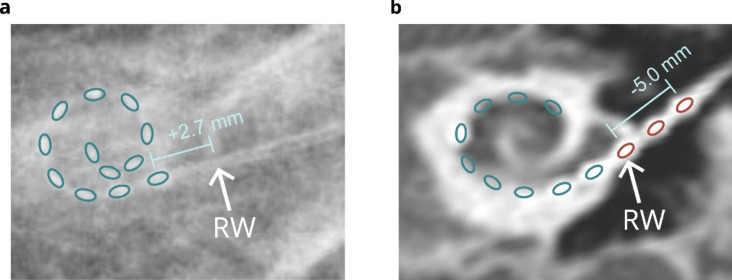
Comparison of postoperative radiographs showing the insertion depth of CI electrodes implanted in the left ear. Linear insertion depth into the cochlea was measured along the electrode array starting from the round window (RW), as indicated by the white arrow. a Plain radiography one day after the surgery. All electrodes are fully inserted into the cochlea, with a linear insertion depth of the most basal electrode of 2.7 mm. b CT scan approx. 22 months after the surgery and before the revision surgery. Three extracochlear electrodes (shown in red) indicate a substantial emigration, with a linear insertion depth of the most basal electrode of − 5.0 mm
